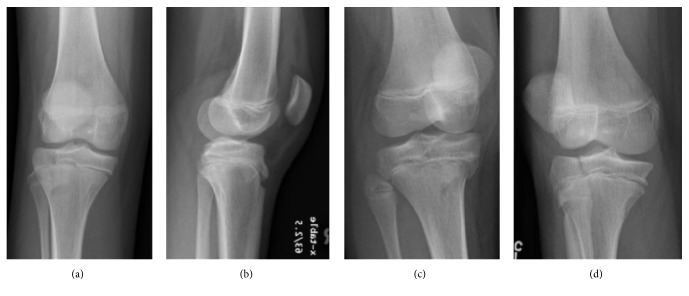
Anteroposterior (a), lateral (b), and oblique (c and d) radiographs of the patient's right knee.
